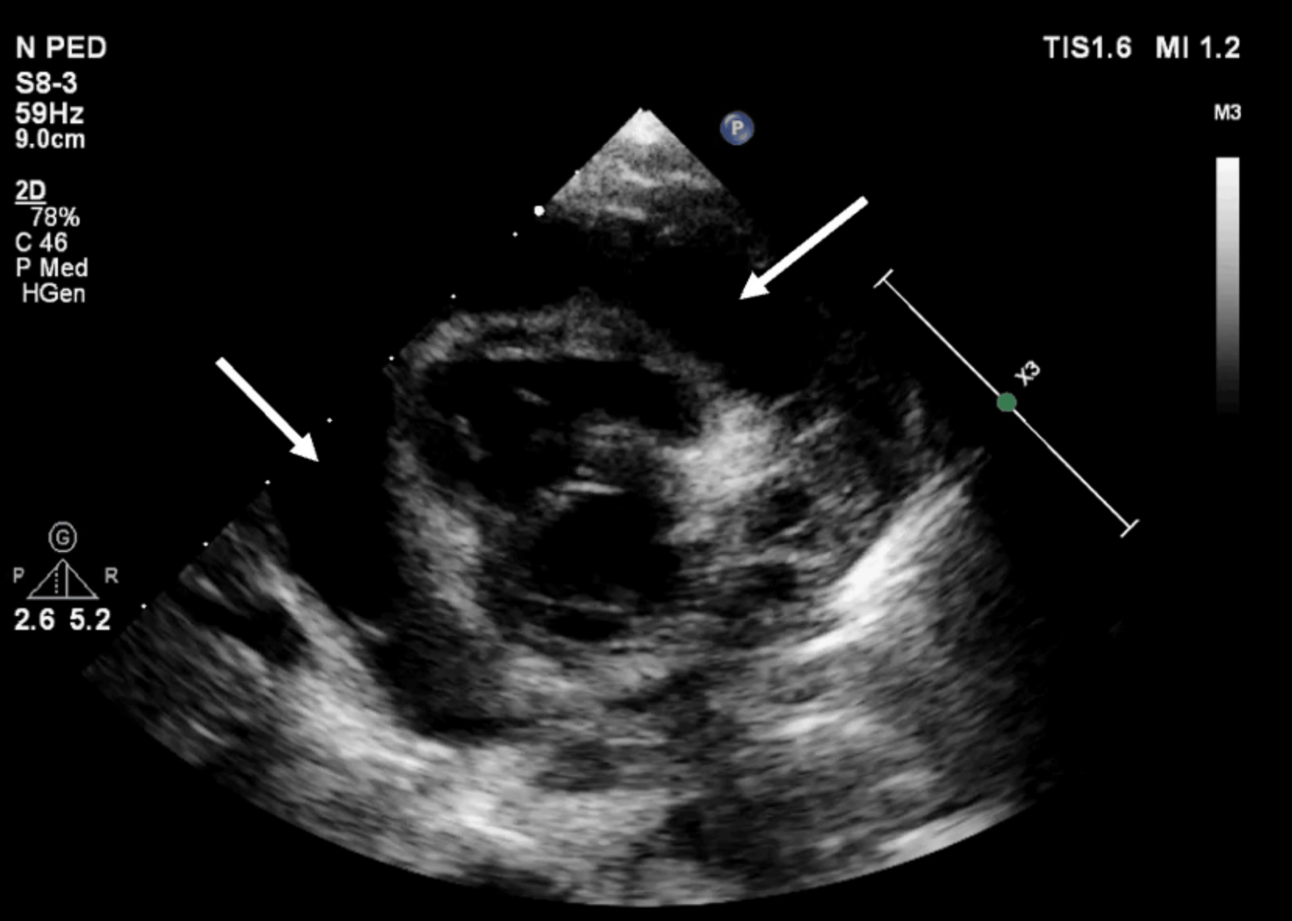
Transthoracic echocardiography (TTE) parasternal short axis 2D view showing circumferential large pericardial effusion (at the time of presentation)
